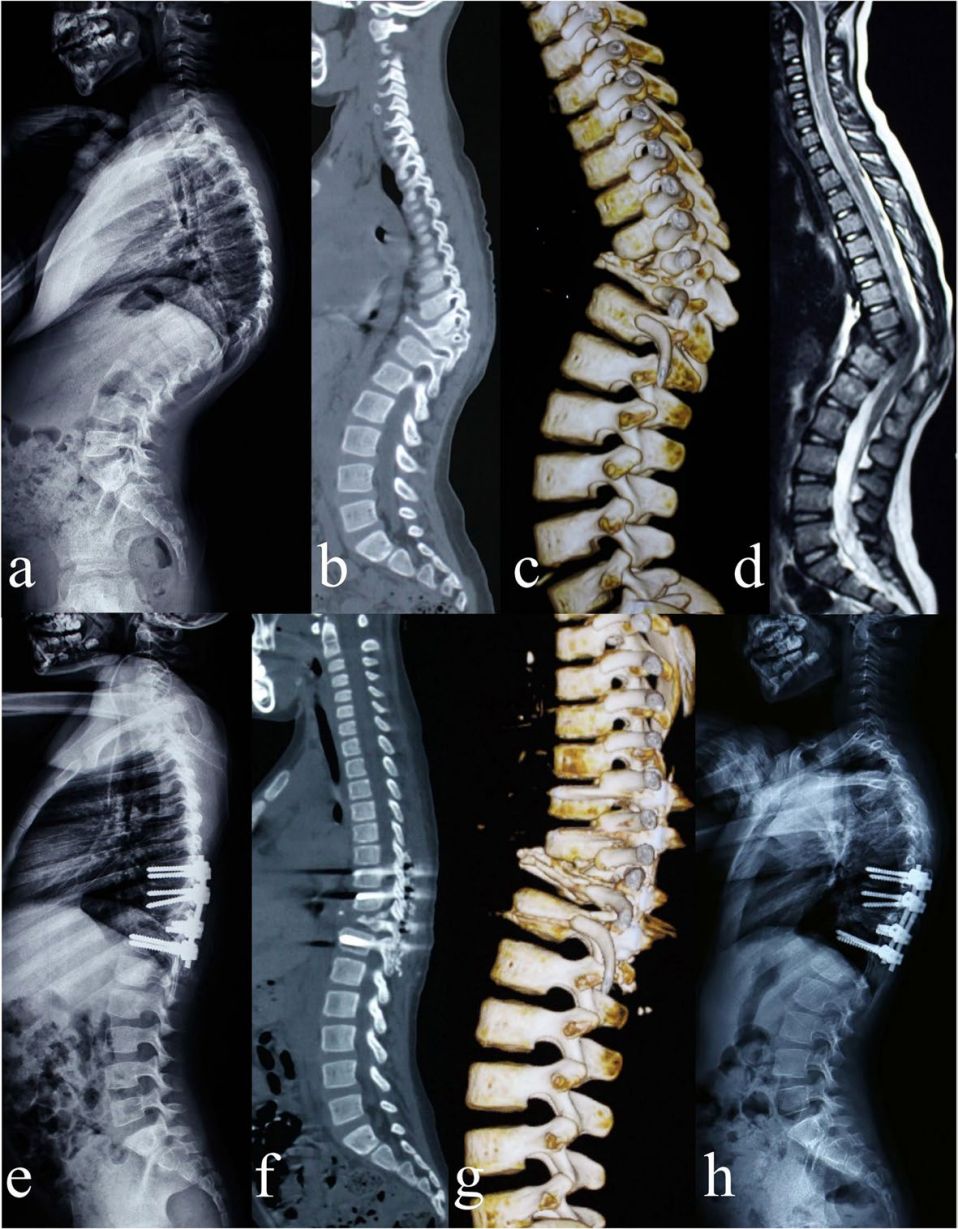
A 5-year-old girl with severe thoracic post-tubercular angular kyphosis. a-d Preoperative X-ray, CT, and MRI showed post-tubercular deformed complex vertebrae involved with T9-11. The angular kyphosis of deformed complex vertebrae was 81.2°, TK was 91.3°, LL was 85.7°, SVA was 4.3 cm, and the spinal cord was compressed obviously. e–g After DCVO, postoperative X-ray and CT showed that the kyphosis of deformed complex vertebrae was reduced to 20.3° with kyphosis correction of 60.9°, TK was 27.5°, LL was 47.9°, and SVA was 0.9 cm. h Postoperative radiographs at 30 months after surgery showed that the kyphosis of deformed complex vertebrae was further reduced to 18.4°, TK was 29.8°, LL was 55.3°, and SVA was 1.4 cm
